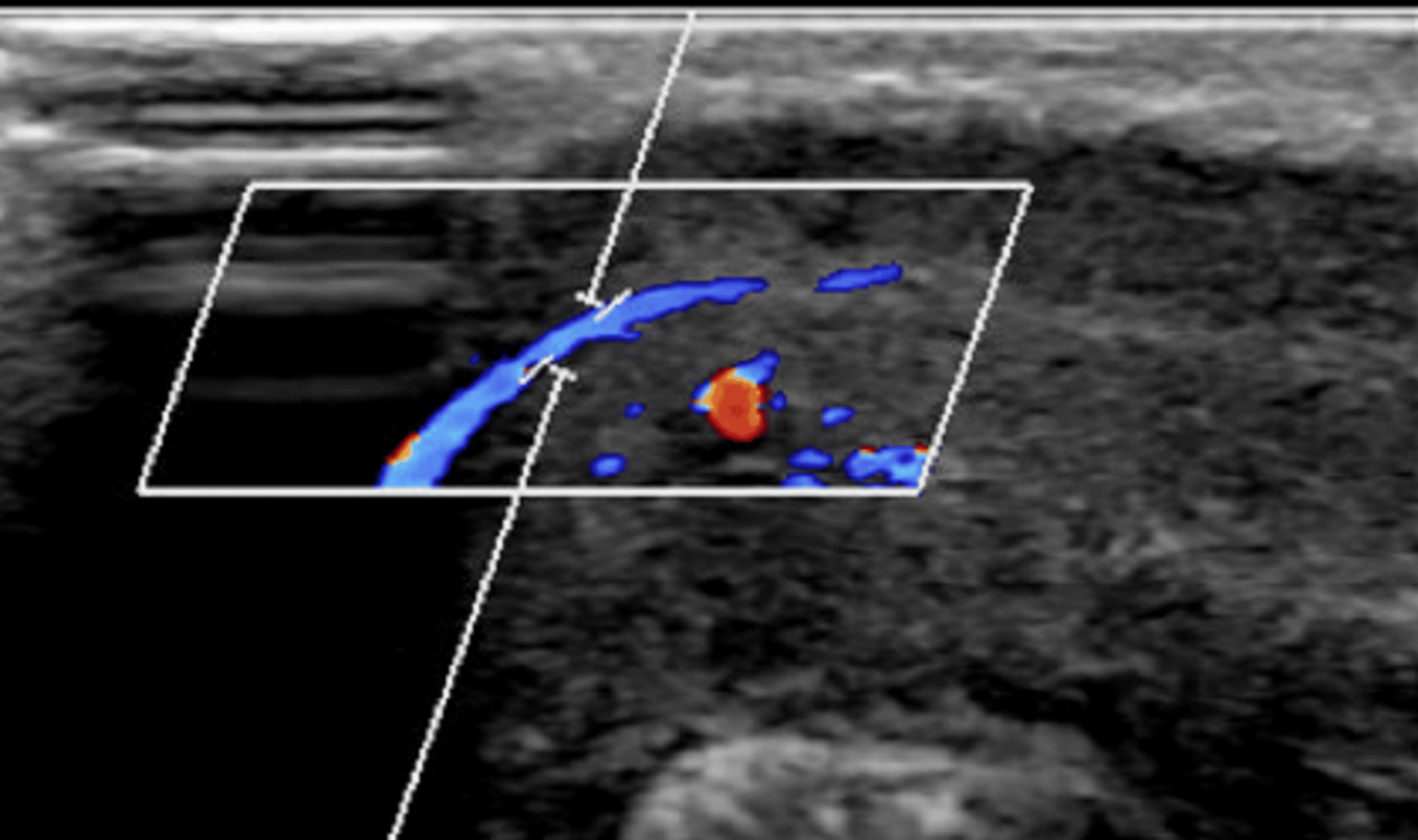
Follow-up duplex ultrasound image at two weeks The previously observed turbulent, high-velocity flow in the right corpora cavernosa has completely resolved. Note that the blue color typically represents venous flow, the red color indicates arterial flow, and the absence of mixed turbulent flow at the injury site confirms the closure of the arteriocavernosal fistula
